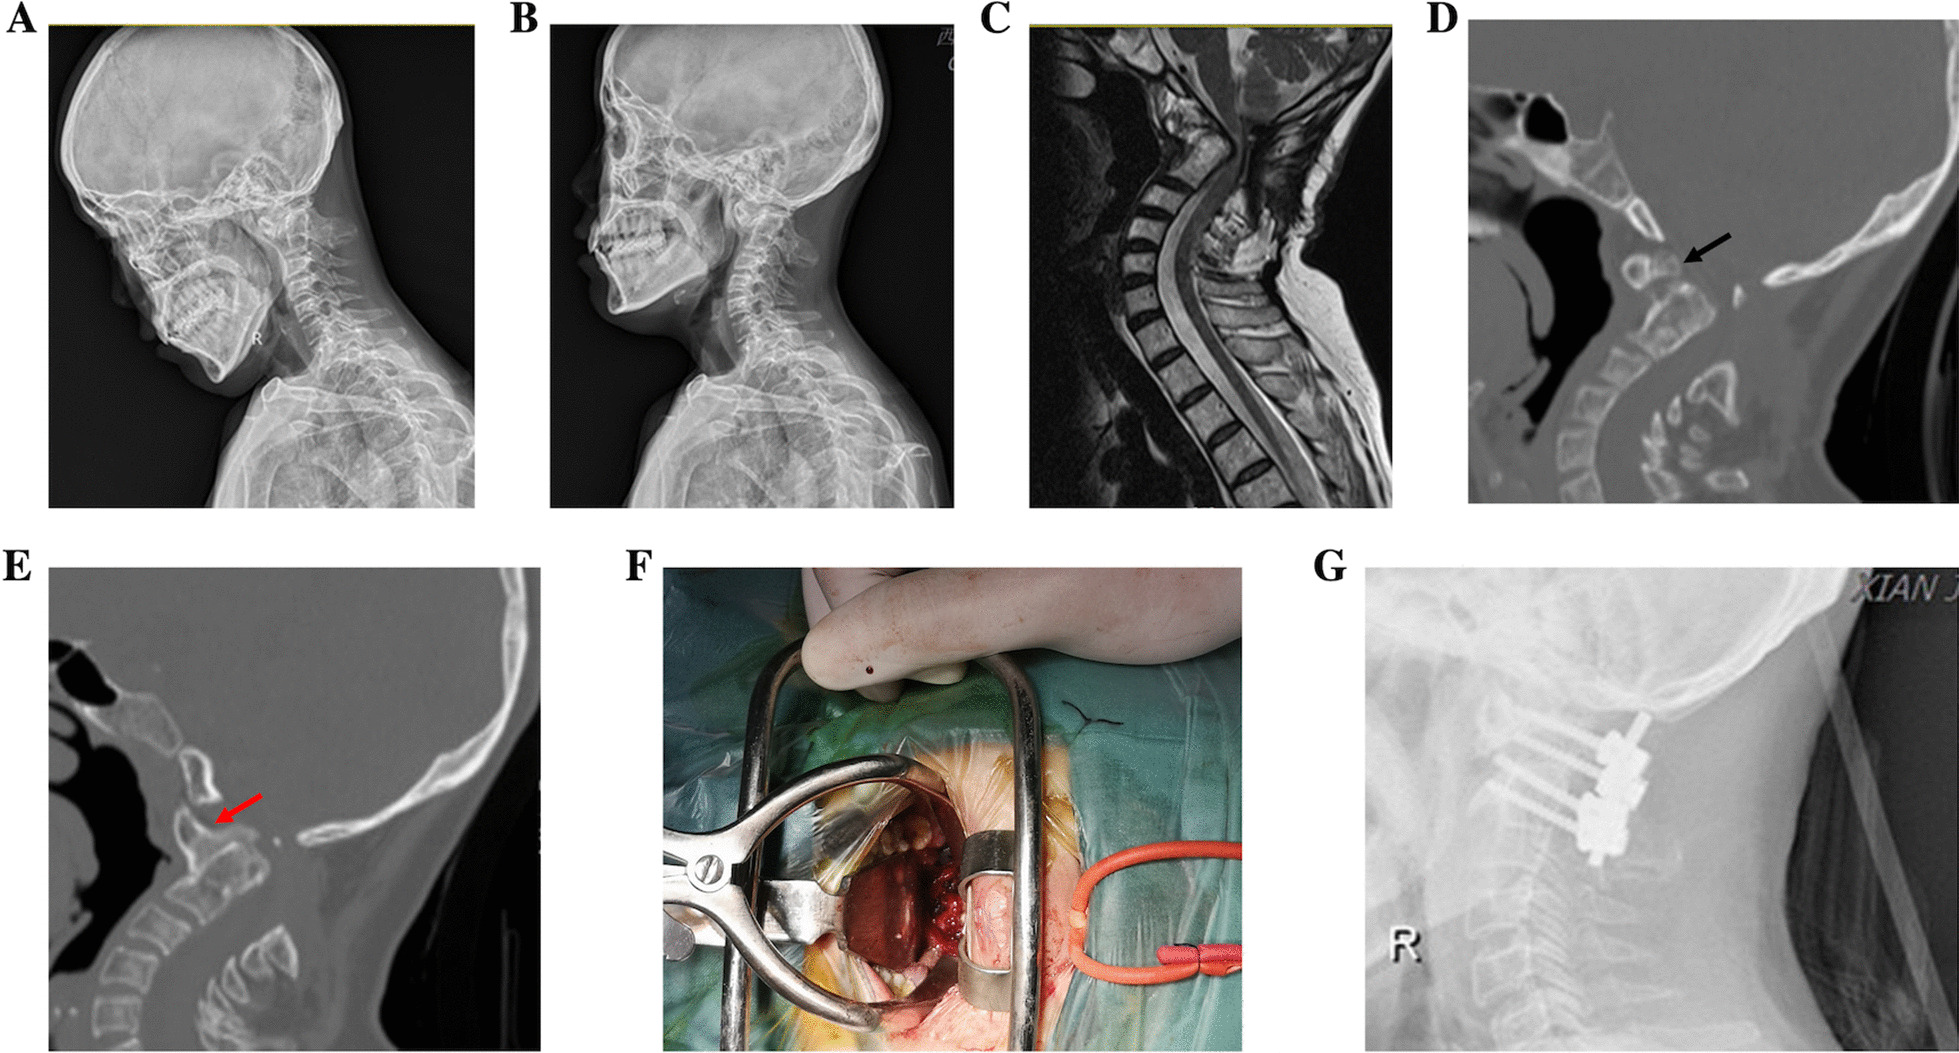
A male patient was admitted for numbness and weakness of limbs for 7 months and rheumatoid arthritis for 5 years. Hyperextension and hyperflexation X-ray presented AAD, and ADI were almost unchanged in dynamic position (a, b). CT showed OO (c, black arrow) and bony fusion between the atlantoaxial joints (d, red arrow), so it was classified as type IV. MRI showed compression of the cervicomedullary junction (CMJ) (e), followed by transoral release (f) and resection of bony fusion tissue. Finally, posterior fixation and fusion were accomplished, and postoperative imaging data showed effective reduction, fixation, and fusion (g)
